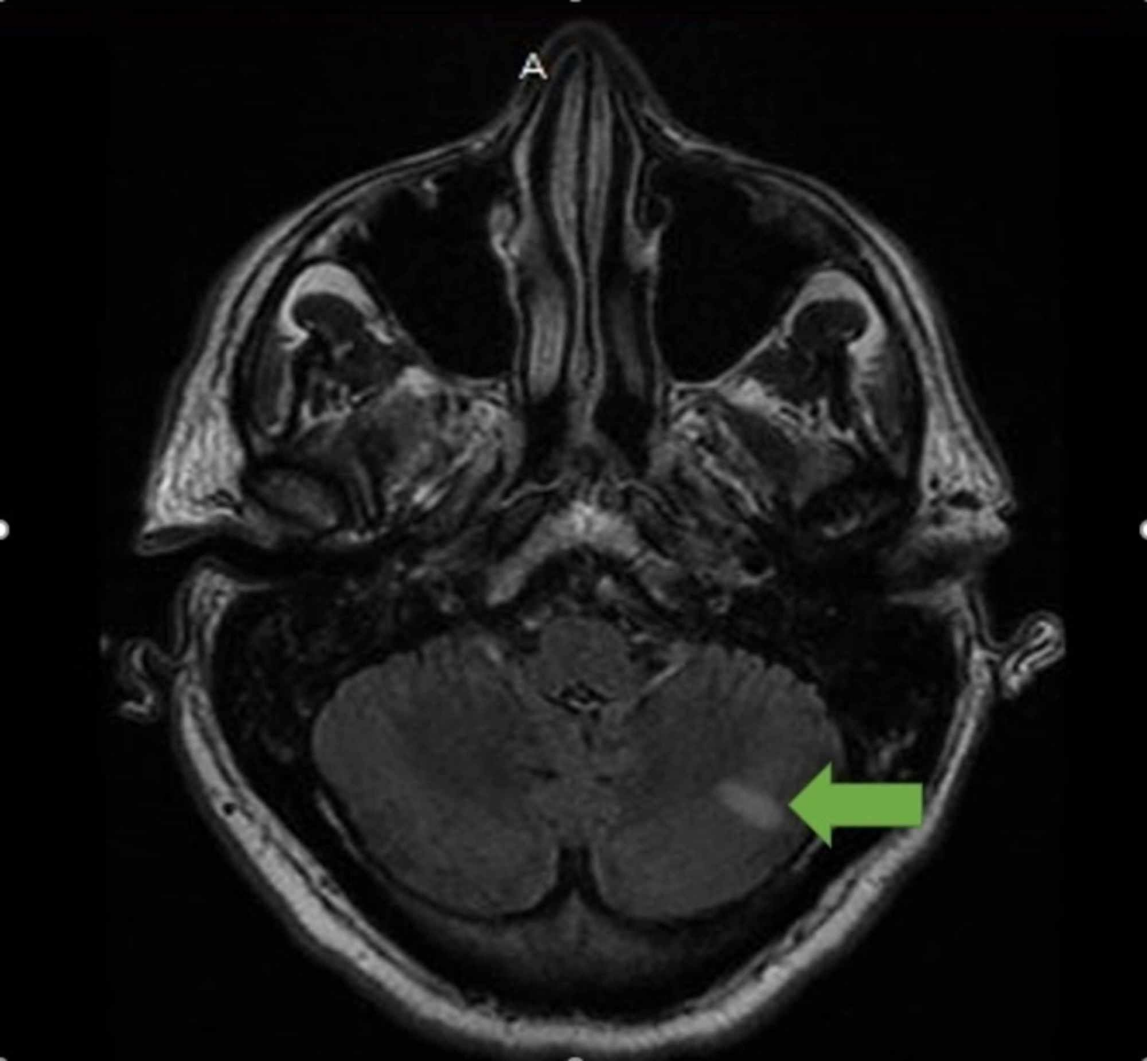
MRI of the brain showing embolic phenomena at the left cerebellum
